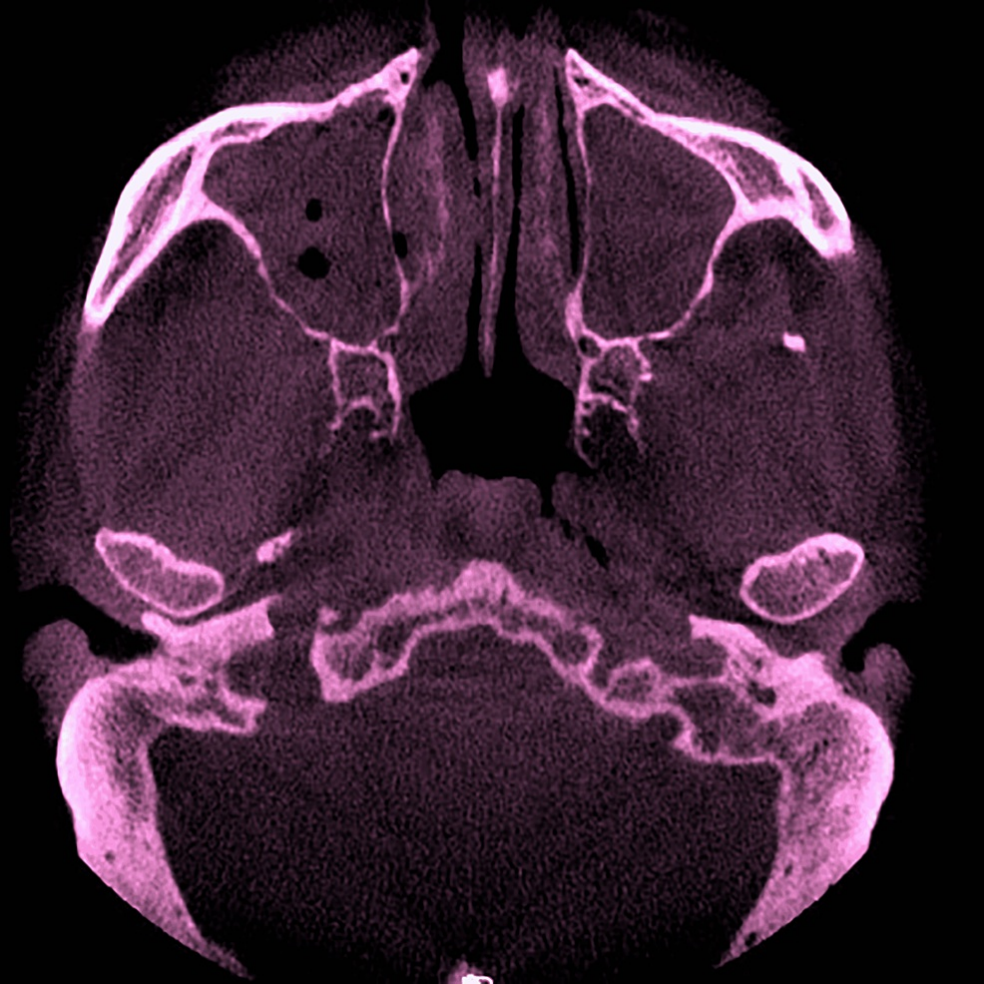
CBCT showing right and left maxillary sinus radio-opacity CBCT: cone-beam computed tomography
